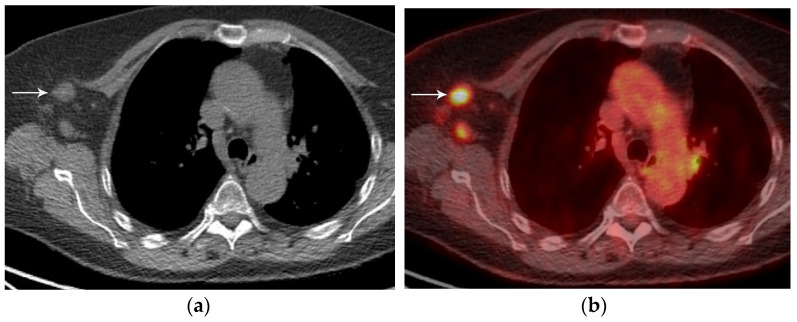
Left lung cancer and right axillary nodal metastases. (a) CT, and (b) axial PET/CT show FDG-avid right axillary adenopathy (arrow). Biopsy confirmed metastatic disease from the left lung cancer. The N status represents regional spread of disease. Lymph nodes not addressed in N classification, such as internal mammary, axillary, and retroperitoneal, represent distant metastatic disease.
